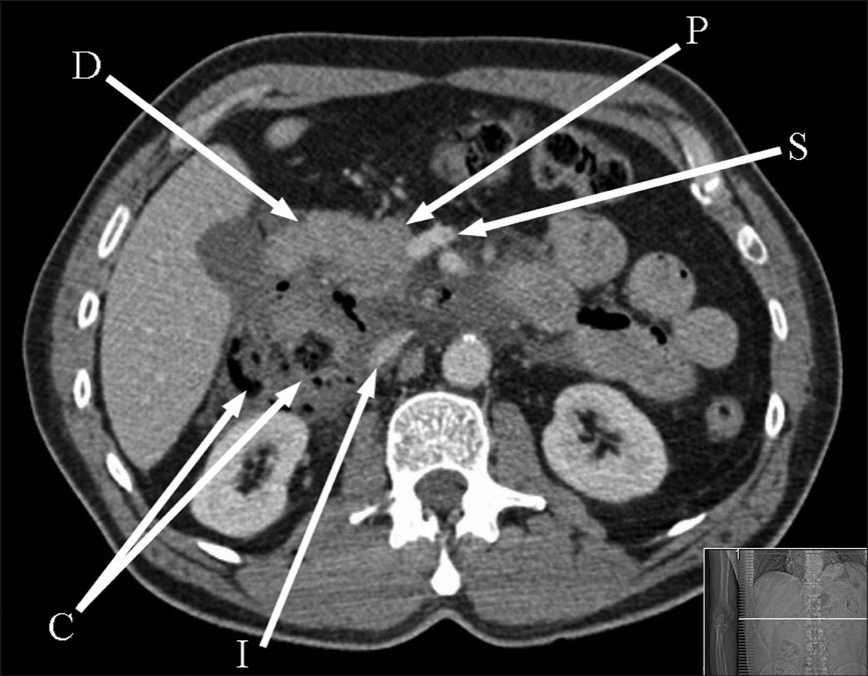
Computed tomography, axial image, demonstrating a retroperitoneal fluid- and air-filled collection posterior to the second part of the duodenum. (P = pancreatic head, S = splenic vein, I = inferior vena cava, C = air- and fluid-filled collection, D = duodenum)
